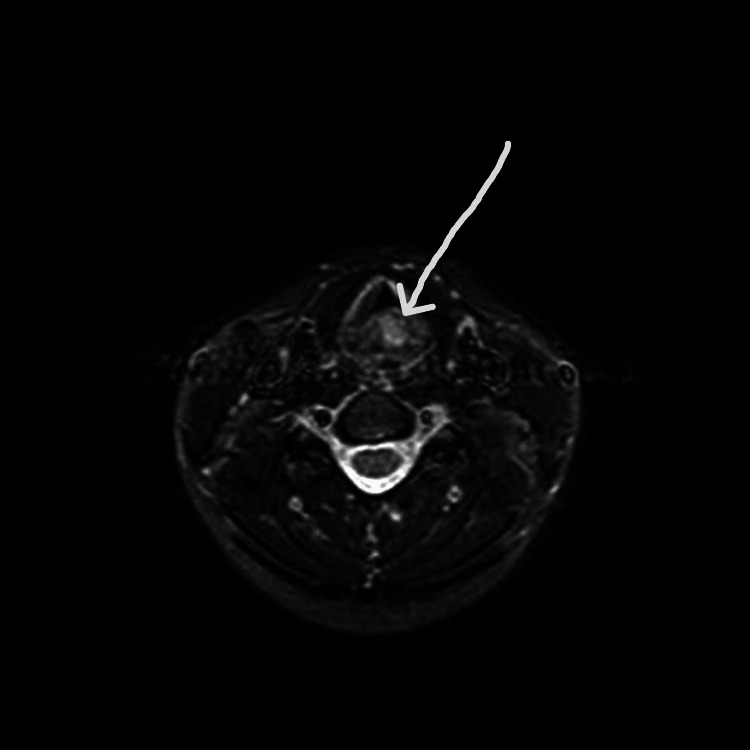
MRI showing hemangioma during the first presentation. The arrowhead is pointing towards the tumor.
